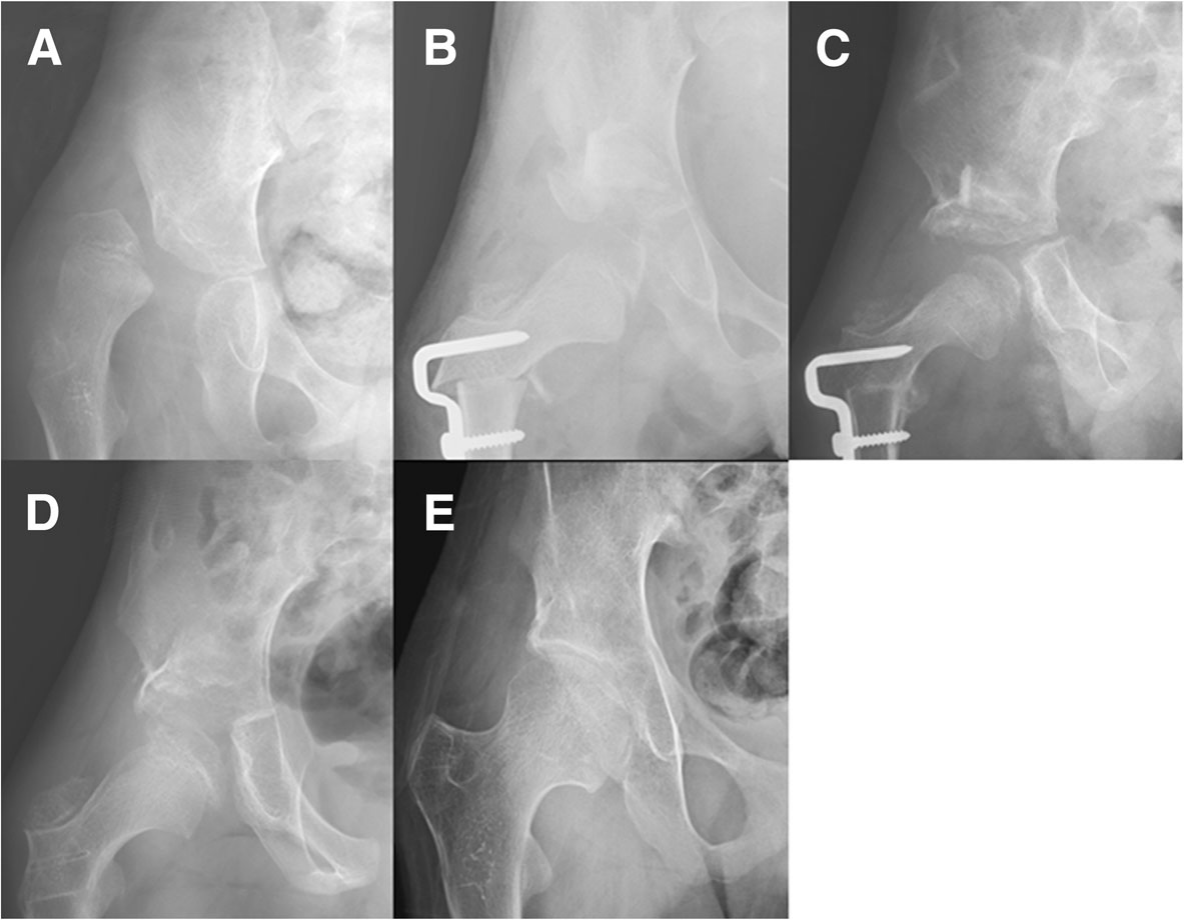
a Preoperative hip radiograph of a 9-year-old body with cerebral palsy shows right hip dislocation and aceteabular dysplasia. b He underwent hip reconstructive surgery including Dega osteotomy using iliac crest allograft for right hip. c The osteotomy site was not incorporated yet at 6 months after the surgery (Goldberg score of 5), d It was completely incorporated at 1 year after the surgery (Goldberg score of 7). e Correction of acetabular dysplasia remained stable at 10 years after the surgery
